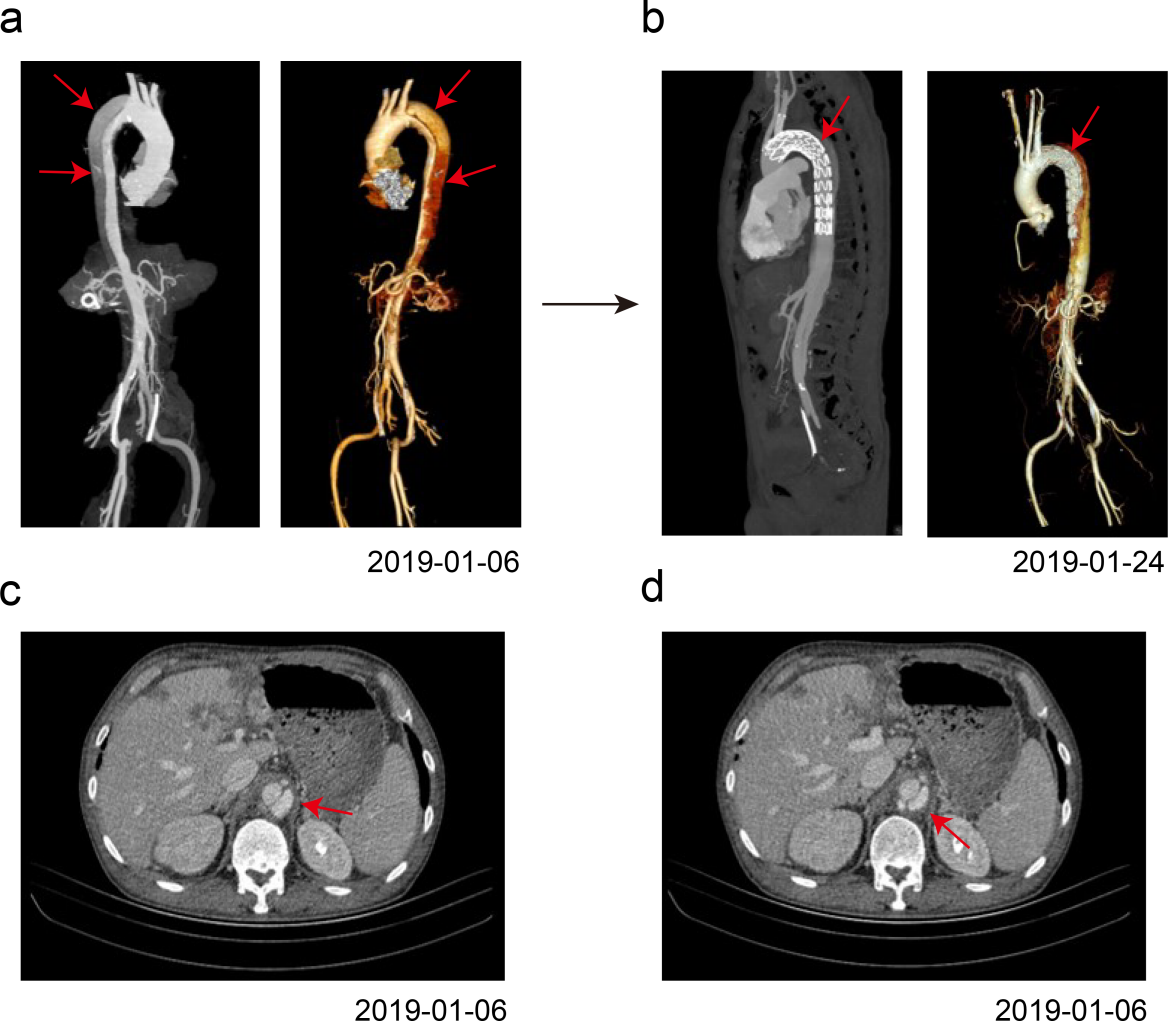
Computed tomographic angiography scan after anlotinib therapy (a) aortic dissection (DeBakey type IIIb), (b) stent‐graft implanted into the aorta; (c, d): thrombosis of the distal false lumen is observed.
